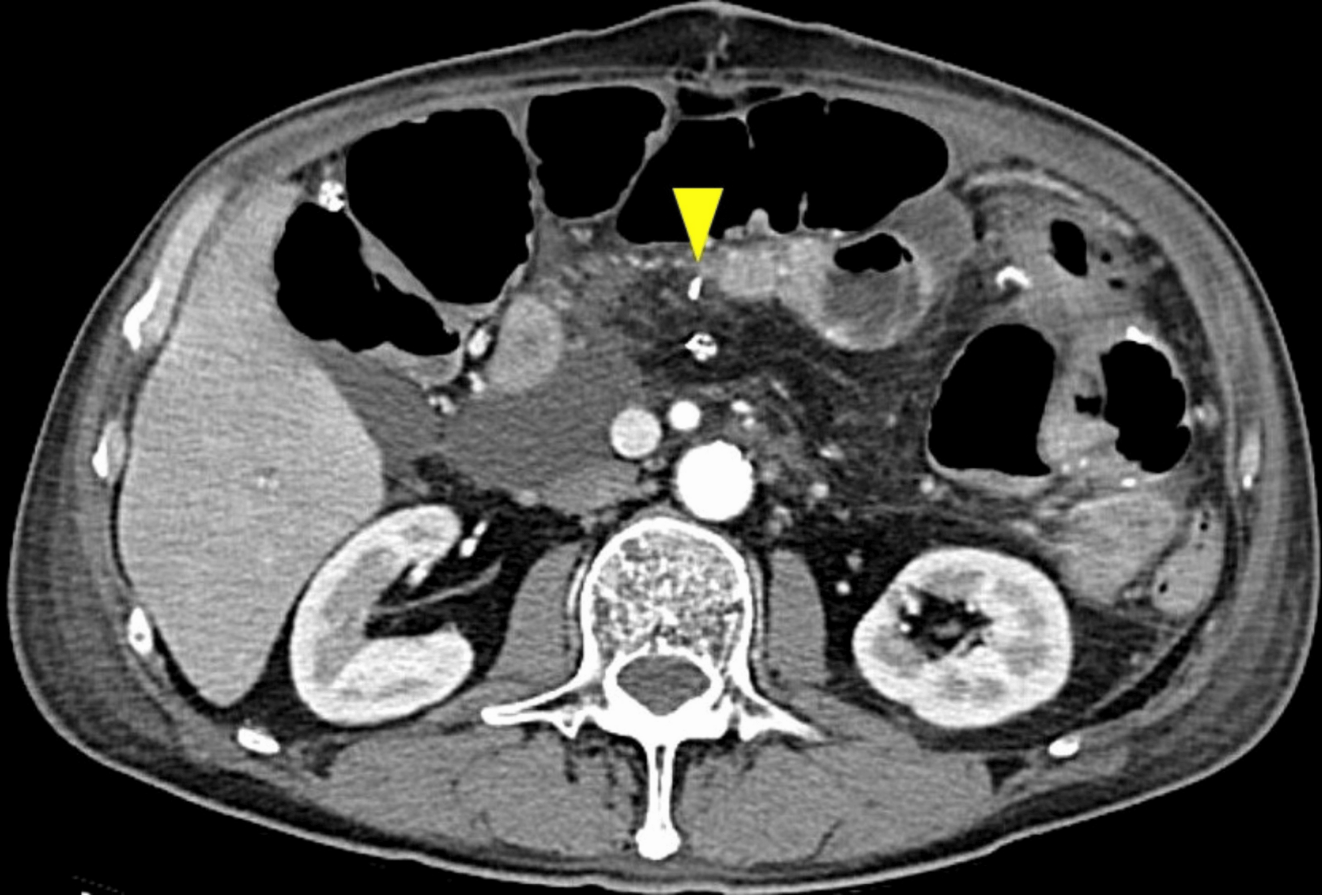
Enhanced abdominal computed tomography of Case 3. Computed tomography showed the pancreatic stent penetrating the jejunum (arrowhead).
